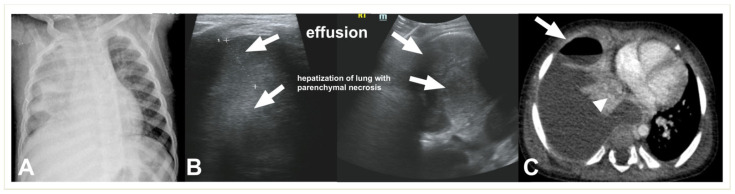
Complicated pneumonia in a 1-year-old boy. (A) Chest radiograph shows opacification of the right hemithorax with areas of consolidation and collapse. (B) Lung US shows an echogenic pleural effusion, making it difficult to distinguish from adjacent echogenic and necrotic lung parenchyma (C). Post-contrast CT scan images depict a large effusion, a loculated air–fluid level in the pleural space (thick arrow), and adjacent collapsed–consolidated lung parenchyma with necrotic areas (arrowhead).
